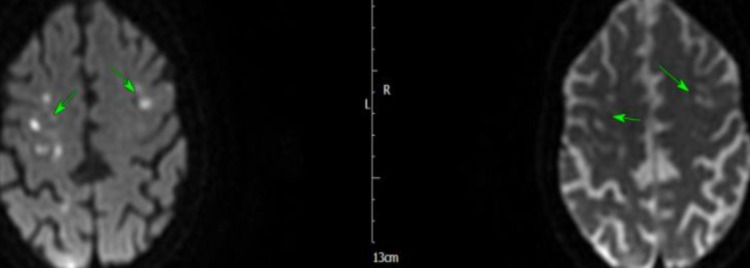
MRI of the head showing subarachnoid haemorrhage (arrows)
